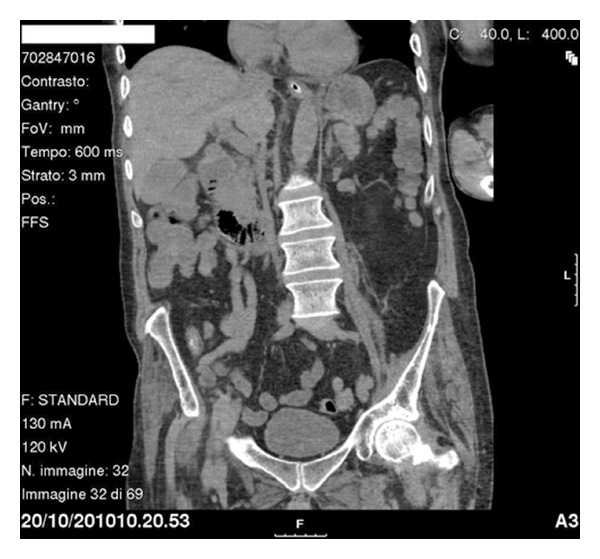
Coronal CT image showing duodenal clamping.
